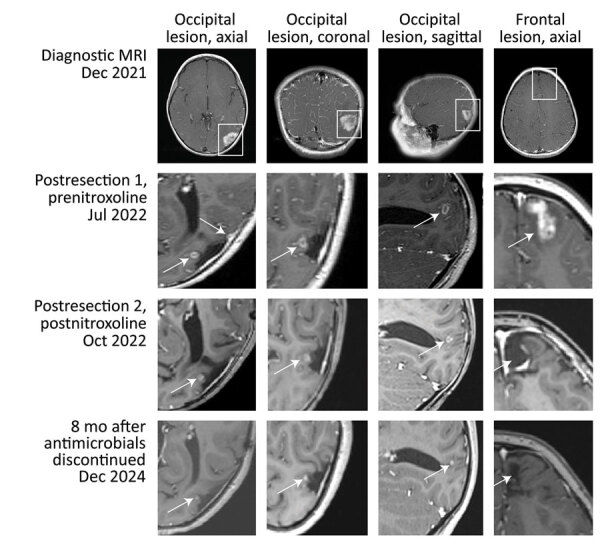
Axial, coronal, and sagittal postcontrast T1-weighted MRI images from study of neurosurgical biopsy and resection for diagnosis and treatment of *Balamuthia mandrillaris* amebic encephalitis, United States. Top row images show patient’s primary occipital and frontal lesions (boxes). Three weeks after resection of the first occipital lesion (second row), recurrent nodules (arrows) developed around the resection cavity. Before nitroxoline initiation, those nodules were enlarging. MRI in July 2022 (second row) showed a new, 7-mm diameter, indistinct, fluid attenuated inversion recovery–hyperintense, enhancing focus in the anterior aspect of the left superior frontal gyrus. After nitroxoline (third row), the occipital lesions retracted (arrows) and were no longer ring-enhancing. No recurrent lesions were noted at the resection site of the frontal lesion, which was resected while the patient was on multidrug therapy and 4 weeks before starting nitroxoline. Follow-up imaging 8 months after antimicrobial treatment was discontinued (fourth row), a stable, residual nodule (arrows) in the medial resection cavity remained, thought to represent gliosis. MRI, magnetic resonance imaging.
